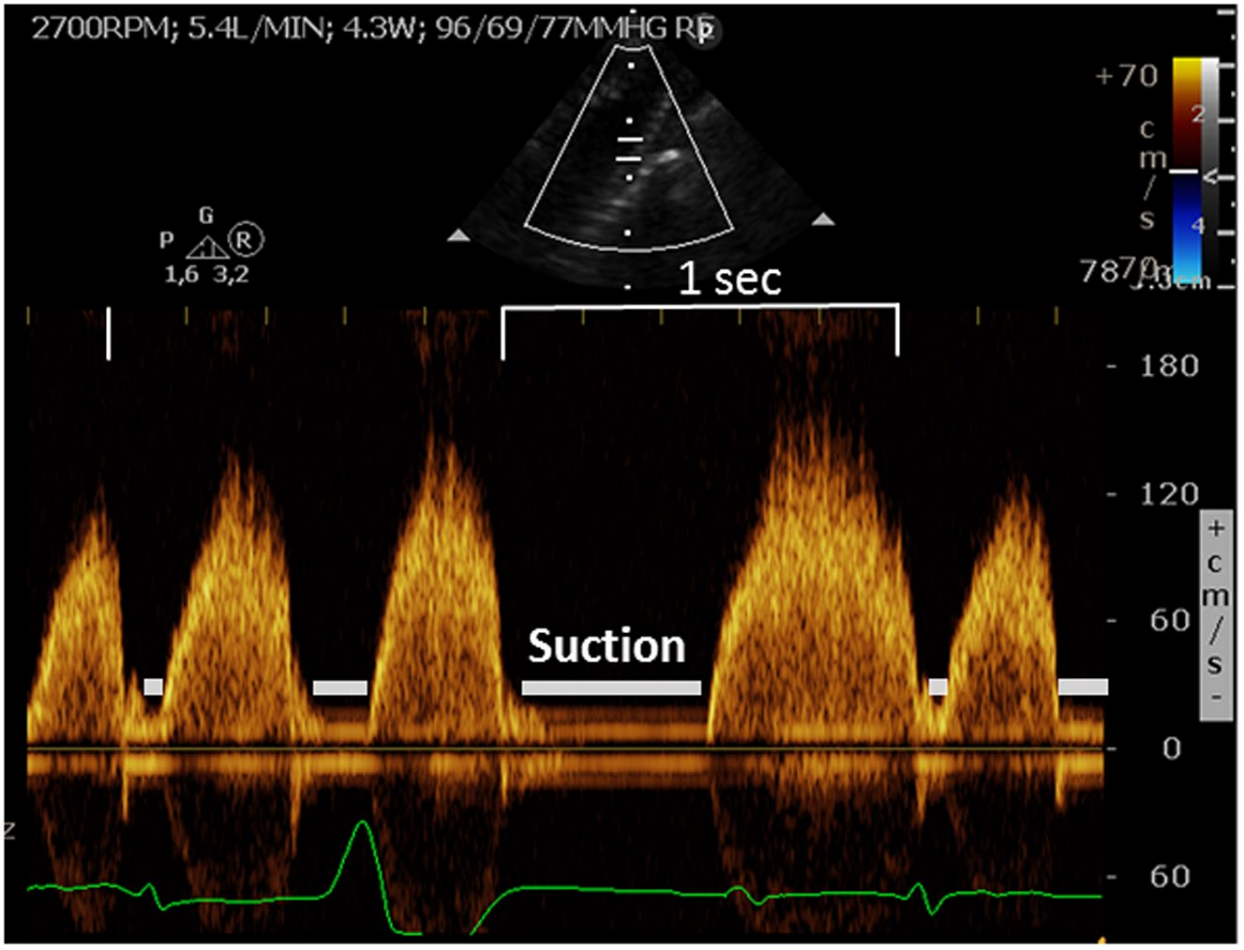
Pulsed‐wave Doppler interrogation of the outflow graft by transthoracic echocardiography in LVAD patient. The spectral Doppler waveform shows abrupt intermittent interruptions of the Doppler signal (marked with white horizontal bars) due to intermittent suction. During these suction events (depicted by the white bars) abnormally low diastolic velocities were measured for the continuous flow LVAD with a speed of 2700 rpm [Color figure can be viewed at wileyonlinelibrary.com]
